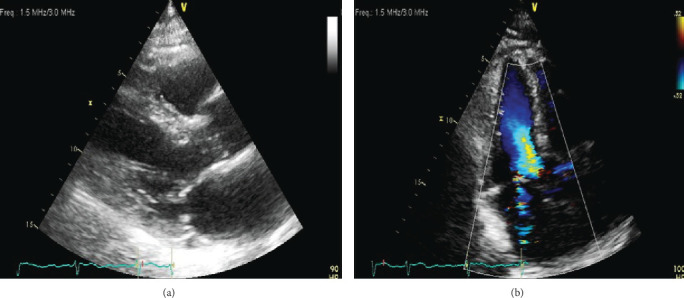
Echocardiograms 25 days prior to leadless pacemaker insertion. (a) A transthoracic echocardiogram (parasternal long) prior to leadless pacemaker insertion showing normal coaptation of mitral valve leaflets. (b) An apical 4-chamber view taken prior to leadless pacemaker insertion showing only mild mitral regurgitation.
